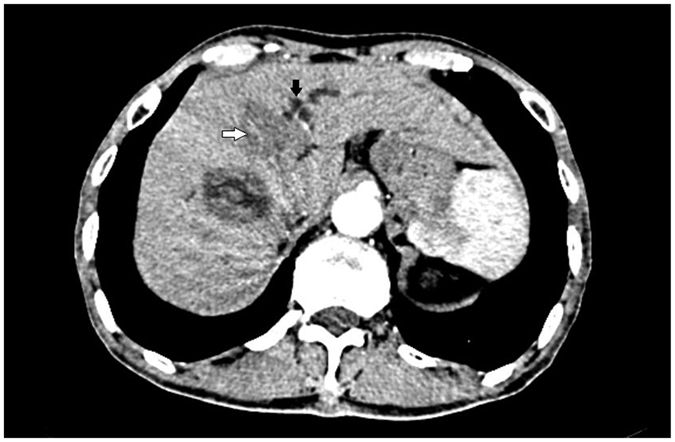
Lesion (white arrow) pushing the left bile duct (black arrow) as shown with enhanced CT.
